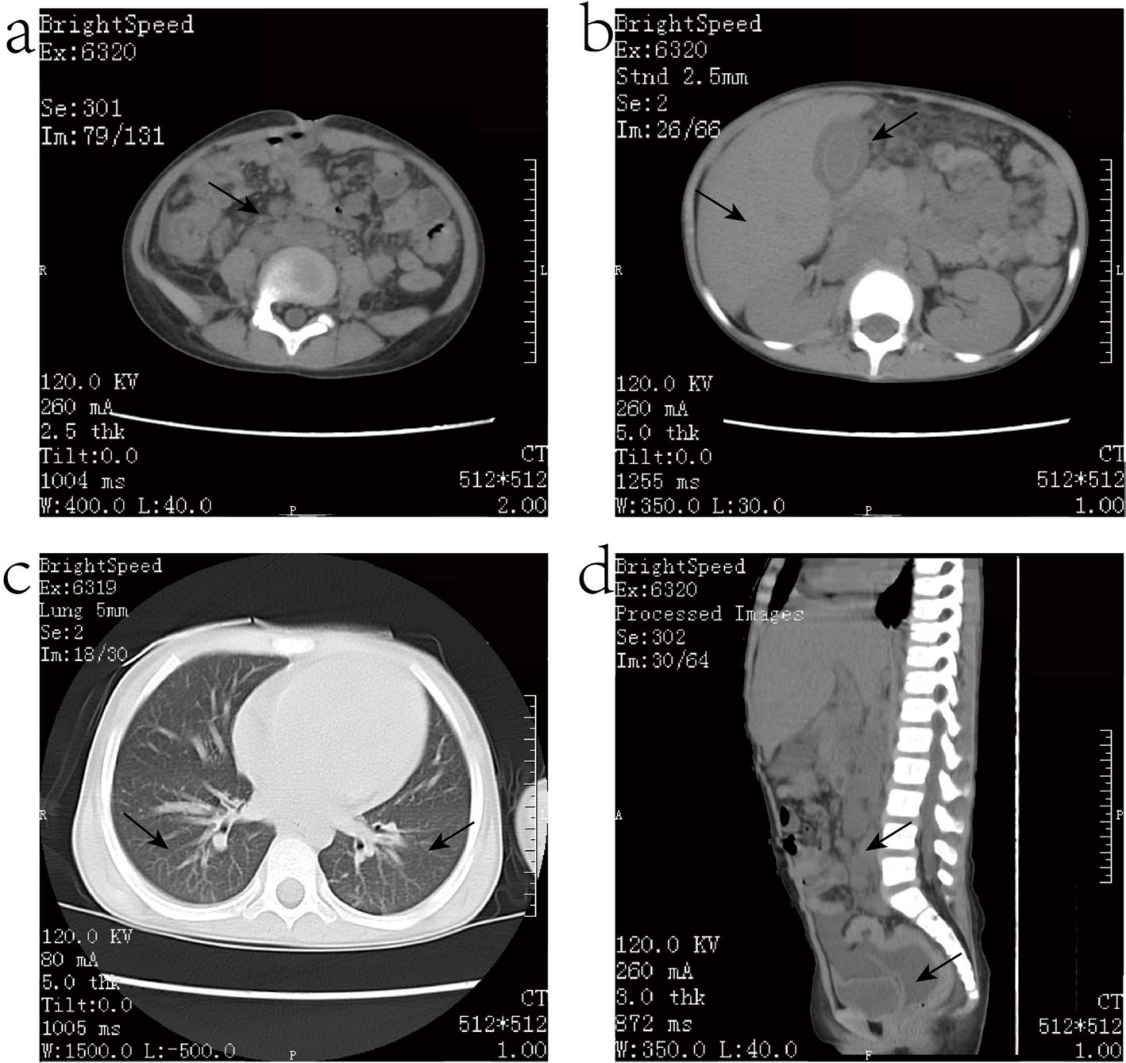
The chest CT manifestations of patient show: 1) multiple enlarged lymph nodes in the abdomen, pelvic cavity, bilateral inguinal region, and axillary region, partially fused into a piece (a); 2) diffuse peritonitis, hepatomegaly, splenomegaly, unclear pancreatic head, cholecystitis with effusion in the gallbladder fossa (b); 3) lower lobe infection of both lungs (c); 4) bilateral pleural, abdominal and pelvic effusion (d)
